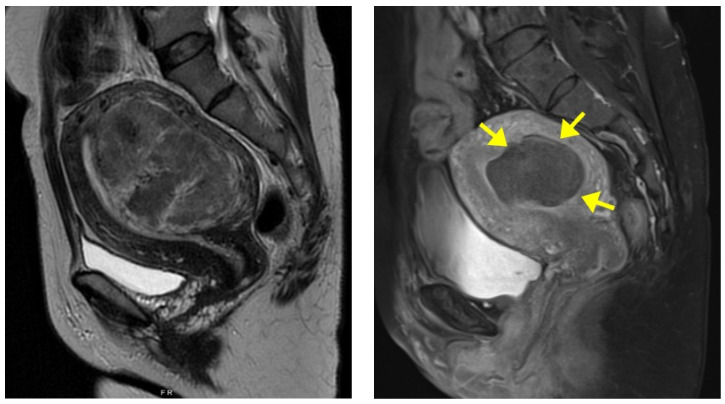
Magnetic resonance (MR) images of a fibroid from a 40-year-old woman; (Left) pre-HIFU MR image showing a large anterior uterine fibroid; (Right) Post-HIFU MR image, 6 months after treatment, showing a hypoperfused well-defined area (arrows) as the result of HIFU with a 73.9% reduction in fibroid volume.
